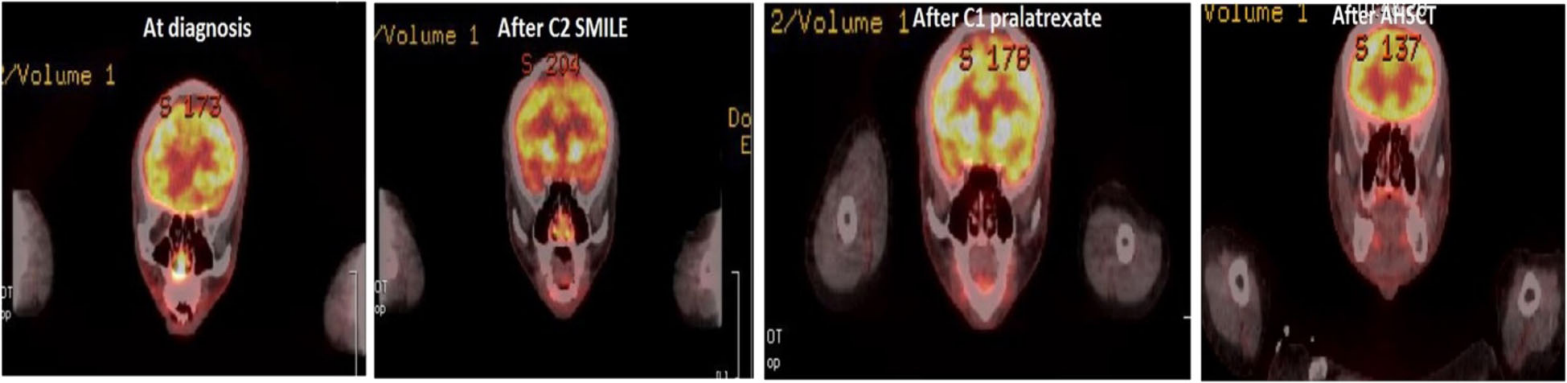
Positron emission tomography-computed tomography scans for nasal lesions and lymphadenopathies showed complete metabolic response after one cycle of pralatrexate and maintained the response after allogeneic hematopoietic stem cell transplantation. *AHSCT* allogeneic hematopoietic stem cell transplantation, *SMILE* dexamethasone, methotrexate, ifosfamide, L-asparaginase, and etoposide
